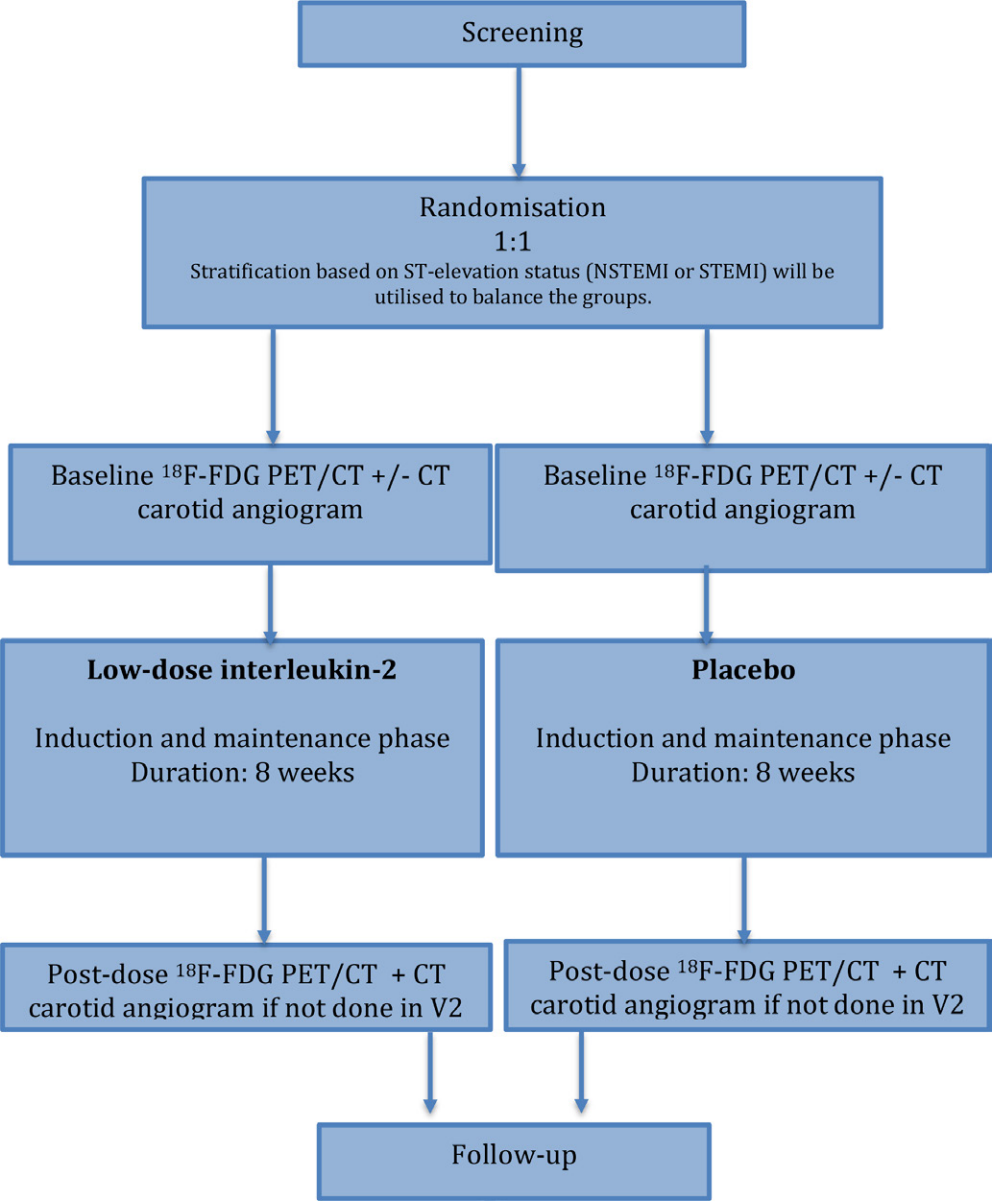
Summary of trial design. ^18^F-FDG, 2-deoxy-2-[fluorine-18] fluoro-D-glucose; NSTEMI, non-ST elevation myocardial infarction; PET, positron emission tomography; STEMI, ST elevation myocardial infarction.
